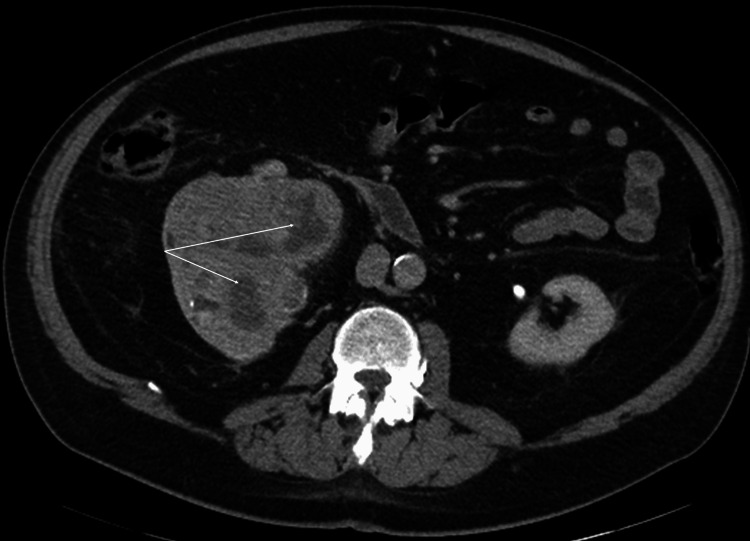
CT urogram CT urogram showed an 11 × 8.3 × 8.4 cm right kidney mass with an extension into the renal pelvis, concerning for aggressive renal cell carcinoma with extension into the renal pelvis or a renal pelvis urothelial carcinoma with extension toward the kidney parenchyma (arrows). CT: computed tomography
